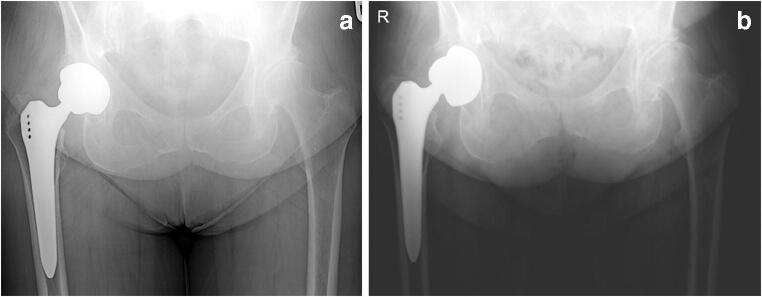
Example of a case with aseptic loosening. a Direct post-operative X-ray. b X-ray at 9 months follow-up with clear loosening of the cup
